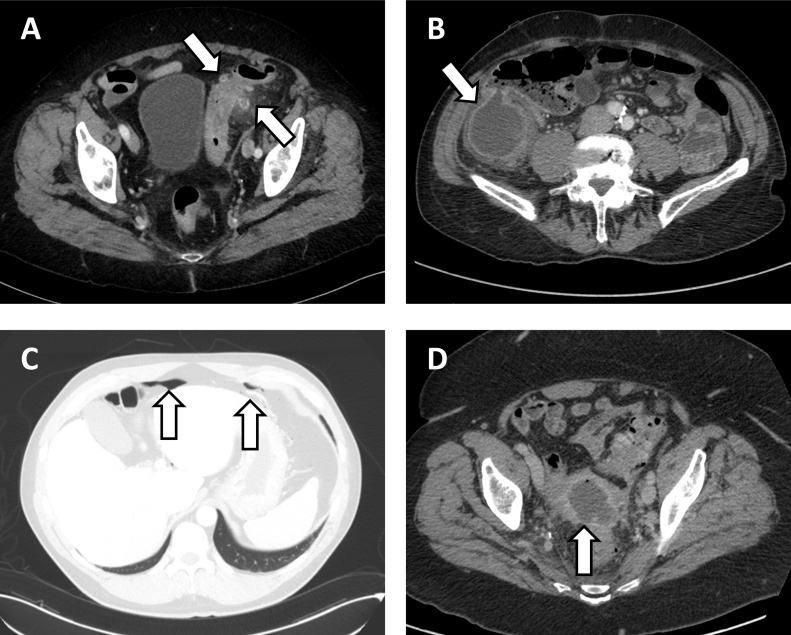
A selection of axial CT images of the abdomen. A) Example of uncomplicated sigmoid diverticulitis. Contrast-Enhanced Computed Tomography (CECT) shows sigmoid colon wall thickening, multiple diverticula and surrounding fatty stranding, suggestive of uncomplicated sigmoid diverticulitis. B) Example of right-sided diverticulitis. CECT shows a large diverticulum originating from the coecum, with surrounding fatty stranding, suggestive of an inflamed diverticulum. C) Example of perforated diverticulitis. CECT shows intraabdominal free air anteriorly beneath the diaphragm, suggestive of perforated hollow viscus. D) Example of complicated diverticulitis with abscess formation. CECT shows a 7 cm large fluid accumulation with a small air-fluid level, peripheral contrast enhancement and surrounding fatty stranding in relation to an inflamed sigmoid colon with multiple diverticula, suggestive of diverticulitis complicated with abscess formation.
